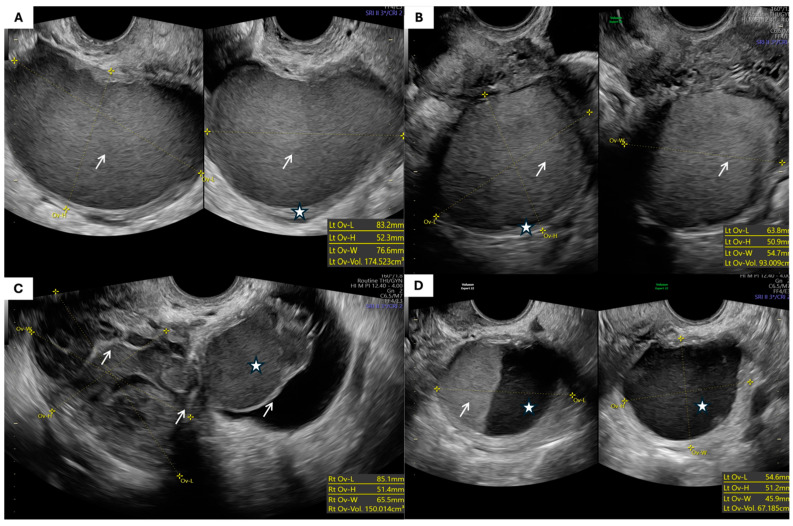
Ultrasonographic appearances of endometriomas: (A,B) Unilocular cyst with ground-glass echogenicity (arrows indicate characteristic ground-glass echogenic content; asterisks mark the thin cyst wall). (C) Multilocular endometrioma (arrows point to septations; asterisk marks the largest locule with ground-glass echogenicity). (D) Multilocular solid endometrioma (arrows indicate solid components and septations; asterisk marks cystic component with ground-glass appearance).
